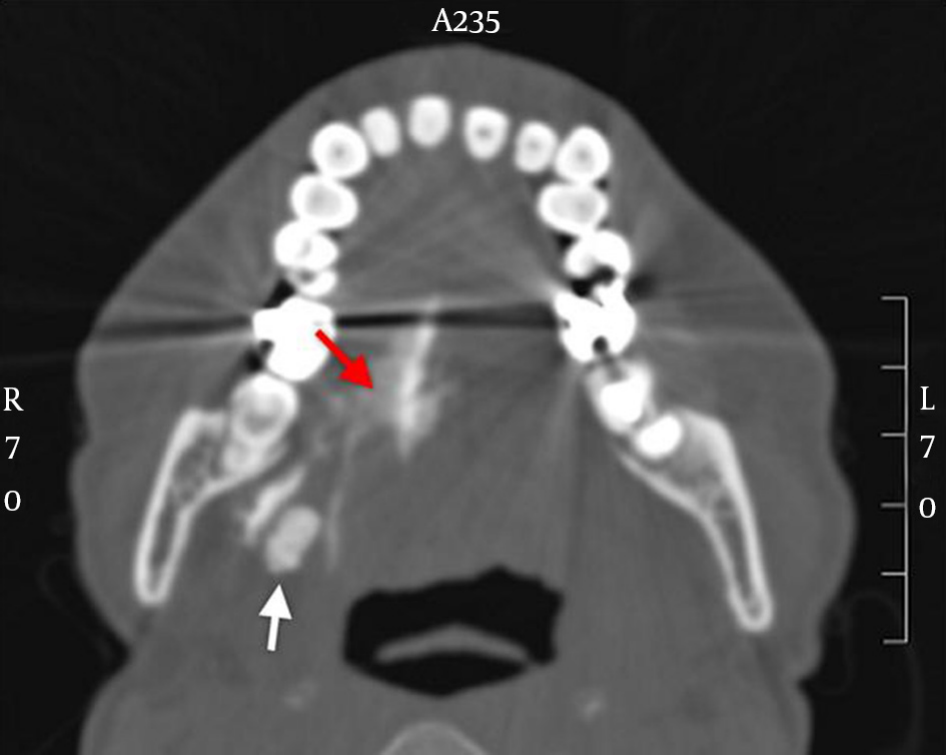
Figure shows a bone window axial cut view of the floor of the mouth CT-scan. A radiopaque submandibular stone (white arrow), and contrast extravasation into the surrounding soft tissues (red arrow) were well seen.
